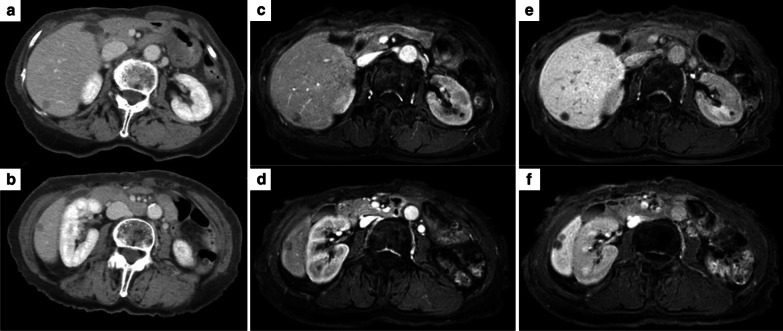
a, b CT image. Three tumors are visible in liver segments S7 and S8. c–f: EOB-MRI image. The tumor edges are hyperintense with dynamic imaging, and some enhancement is exhibited within the tumors, similar to the previous findings
